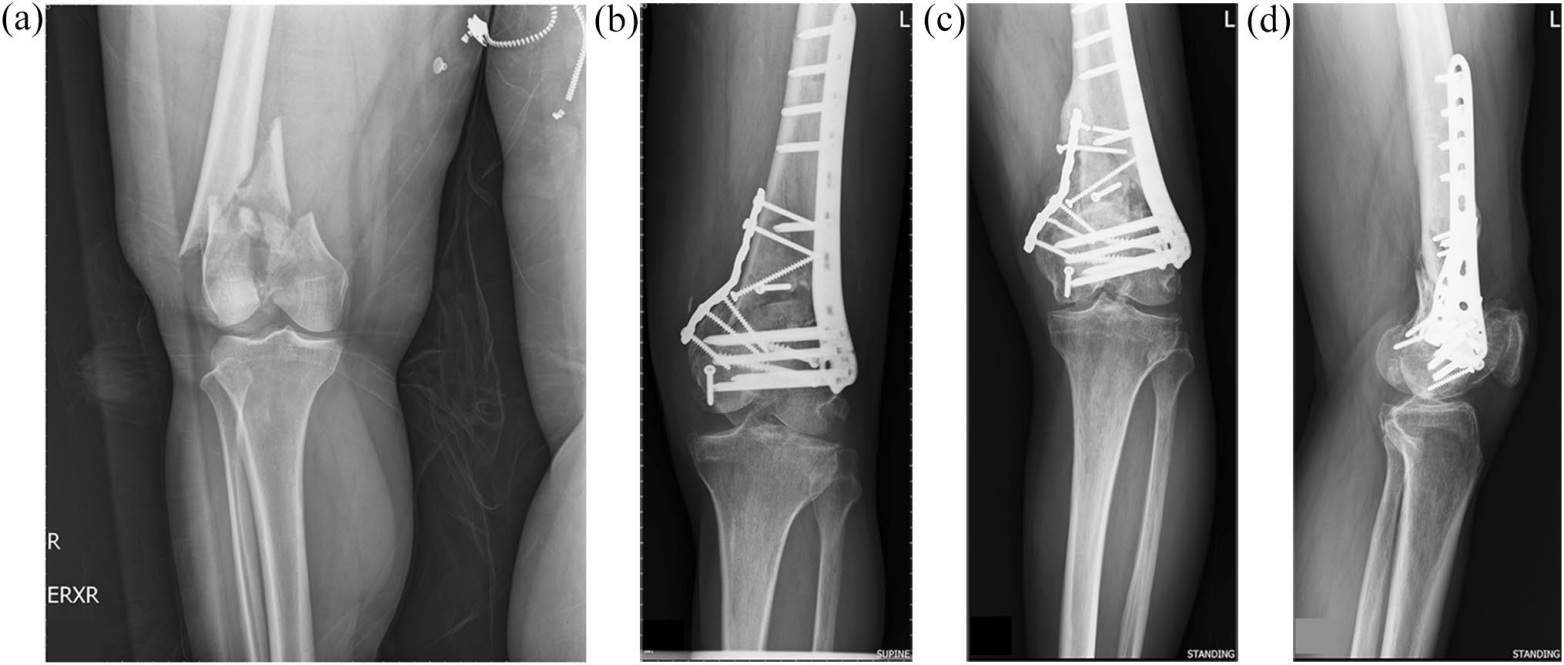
a A 59-year-old male with closed AO type C3 left distal femoral fracture. b Post-operative anteroposterior plain film after osteosynthesis with double plates. c and d The fracture was collapsed and the medial buttress plate was loosed at 21 months. The change of LDFA was 5˚
